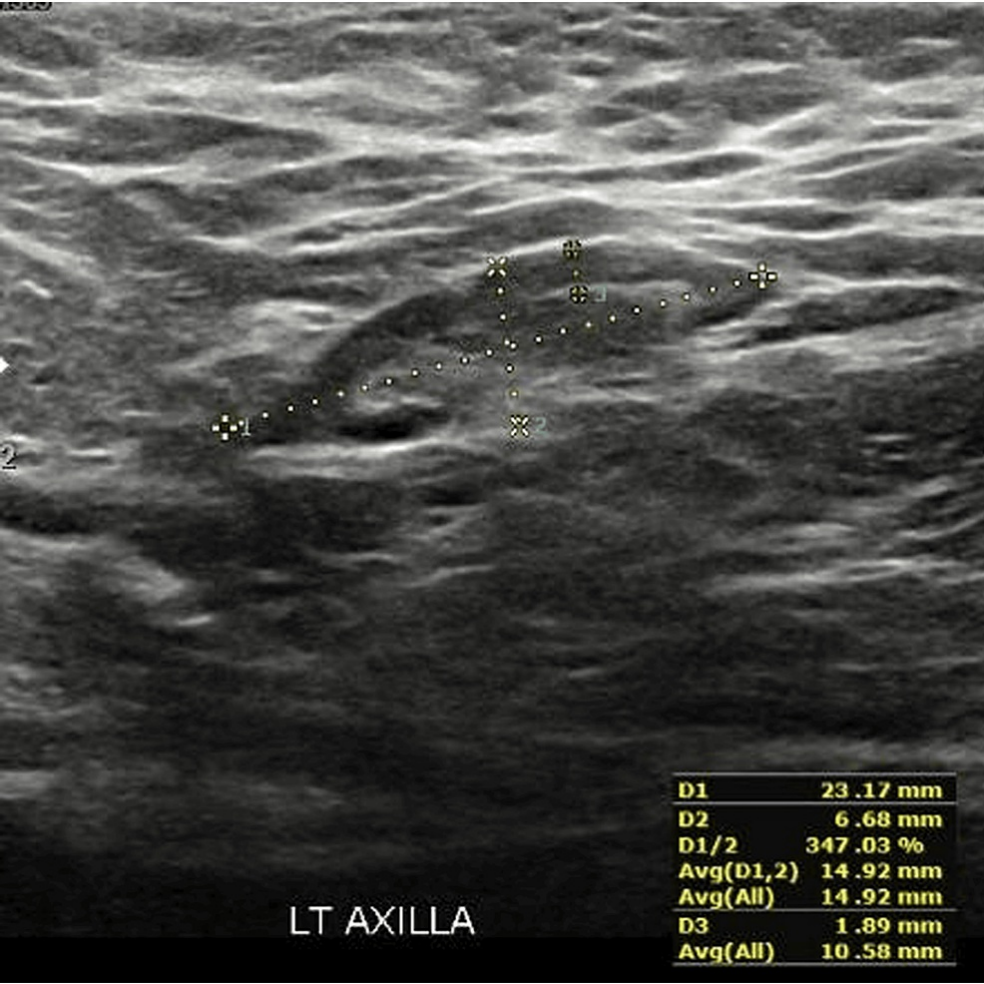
Ultrasound image showing a normal axillary lymph node This figure was obtained from the KFUH system (author's own).
